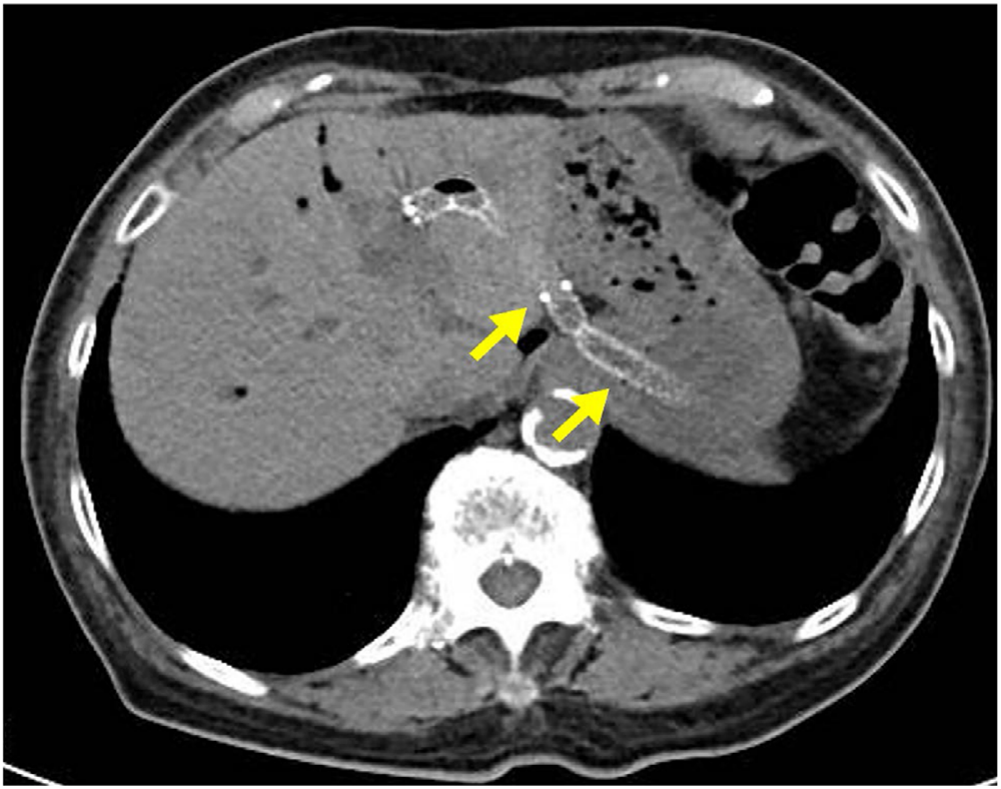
Abdominal computed tomography shows the resolution of the intrahepatic hepatic duct dilation and the placed stent (arrow)
